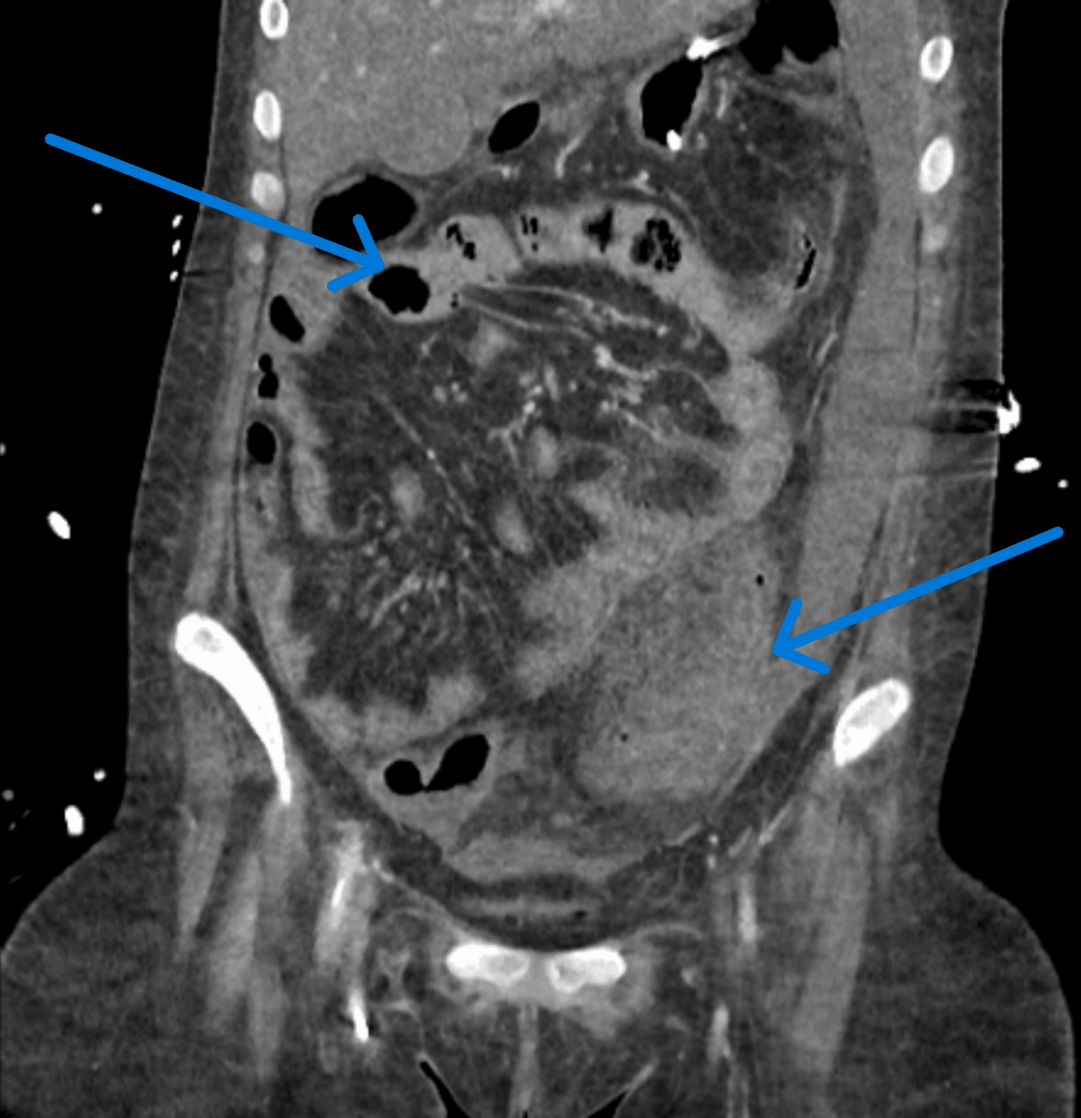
Contrast-enhanced CT scan of the abdomen and pelvis suggestive of inflammatory bowel disease involving most of the colon with ascites, along with adjacent inflammation of the left psoas muscle.
